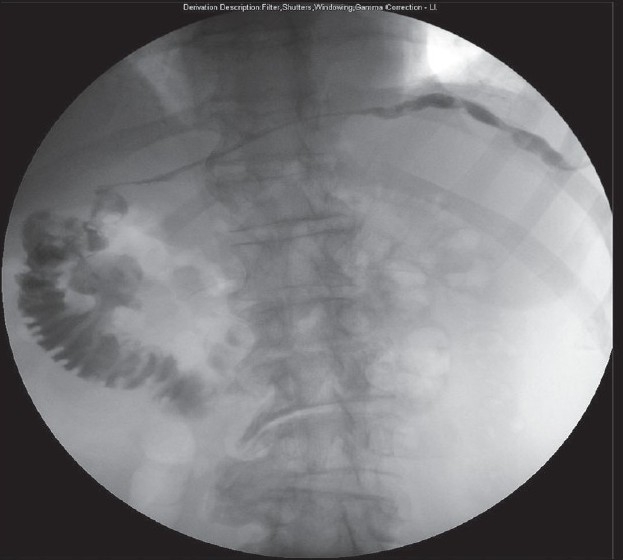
A radiographic sinugram, clearly demonstrating the fistulous tract communicating with the second part of the duodenum
